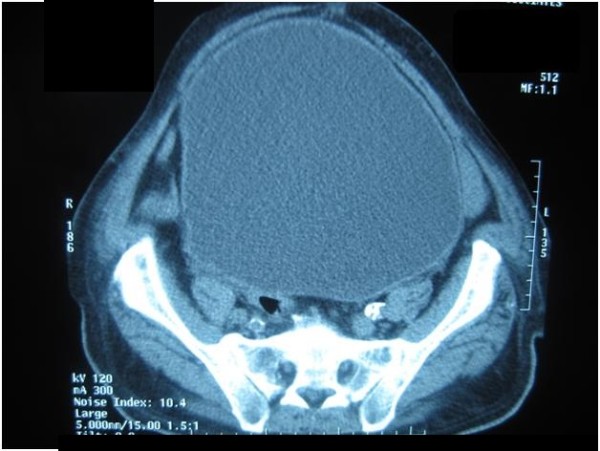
Computed tomography scan of the abdomen showing a distended urinary bladder. Computed tomography image of the abdomen found a grossly distended urinary bladder extending out of the pelvis.
